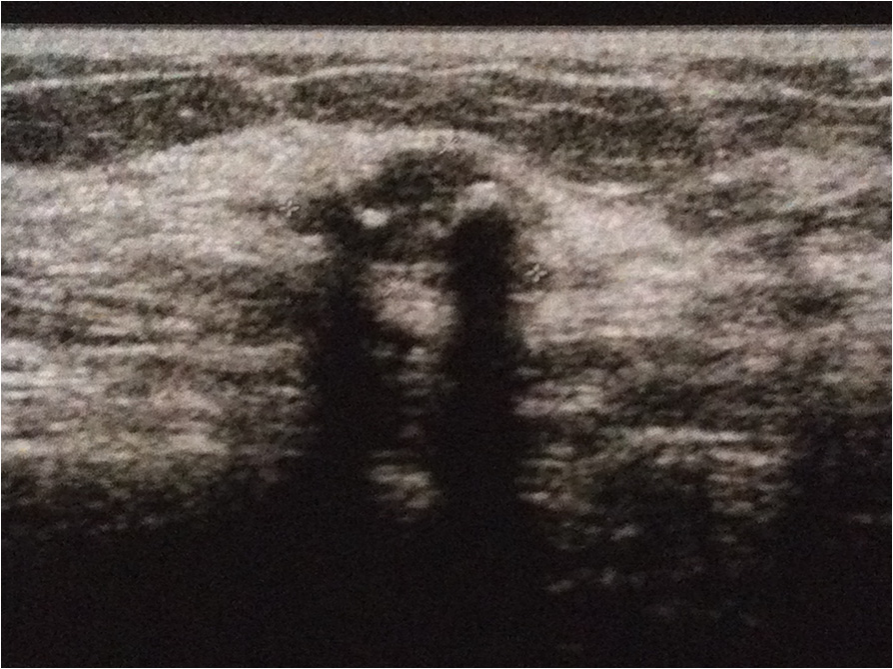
Hypoechoic lump at breast ultrasound.
